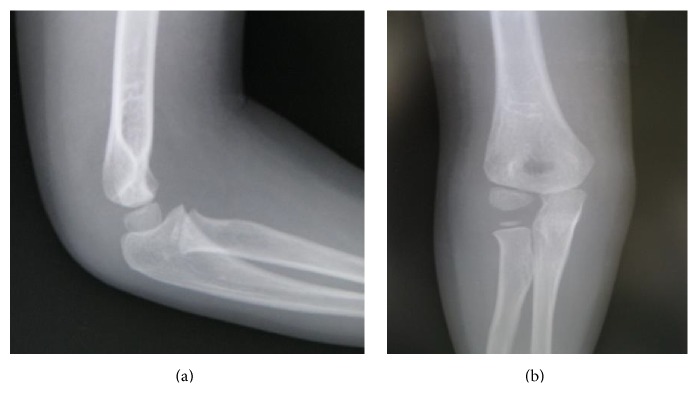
Plain X-rays of the right elbow. There is no sign of osteolysis. (a) Lateral view. (b) Anteroposterior view.
